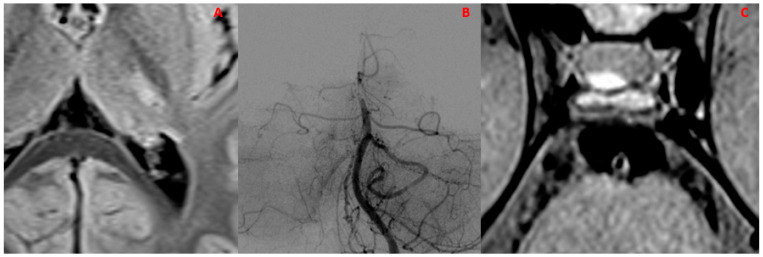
Patient (M/5) with a diagnosis of infectious (VZV-related) CNSV. (A) FLAIR T2-weighted image showing left thalamus hyperintensity suggestive of ischemic lesion. (B) Angiographic image of stenosis of the basilar artery. (C) 3D SPACE T1-weighted VW image shows intense, regular, concentric enhancement of walls of the basilar artery at the site of the angiographic stenosis.
